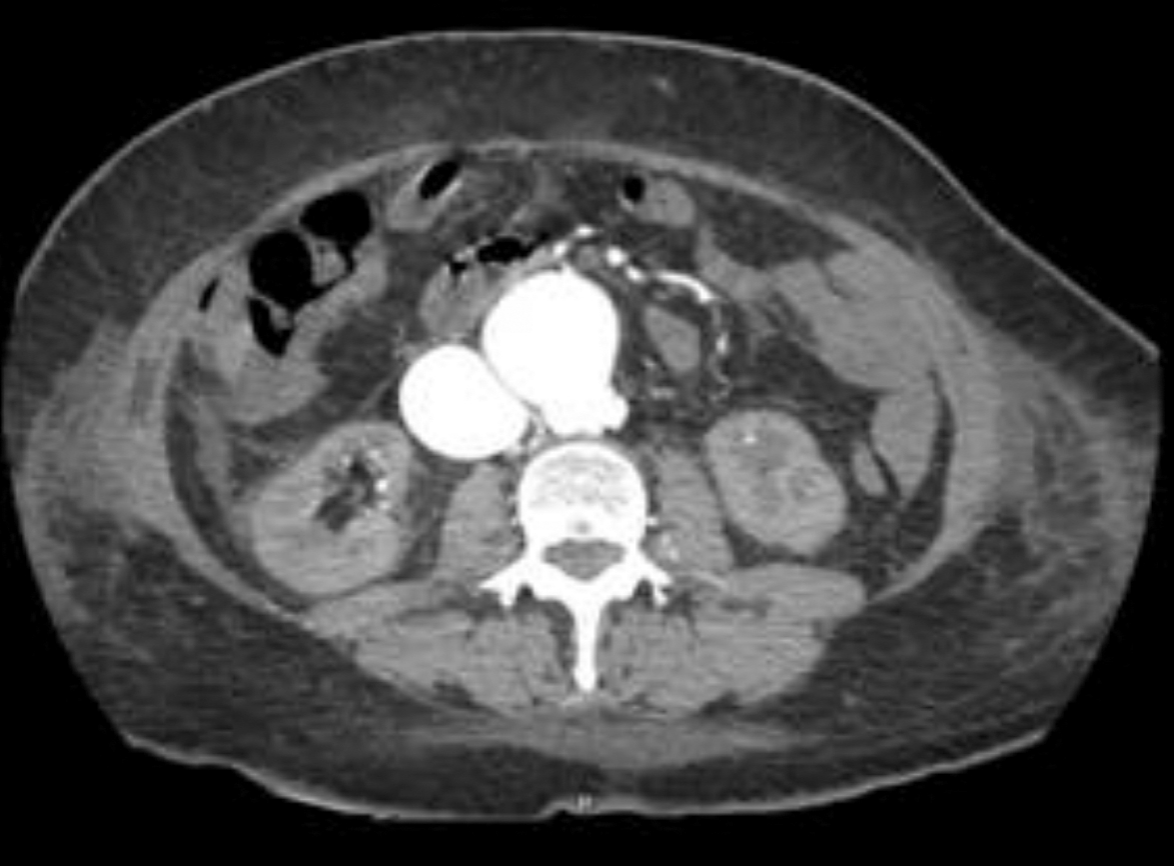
Contrast CT scan at the emergency department confirming the fistula between AAA and the aberrant left renal vein.
